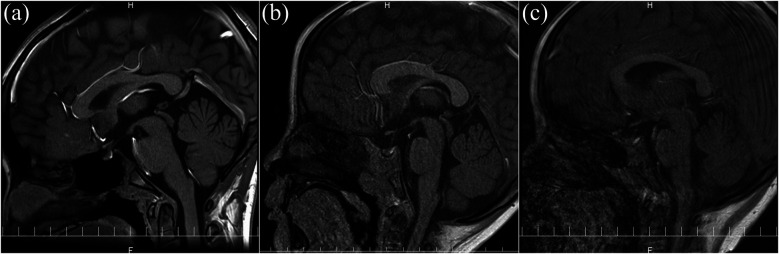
Representative images of the MRI quality: (a) no artifacts and fully evaluable; (b) mild artifacts and evaluable; (c) severe artifacts and not evaluable.
